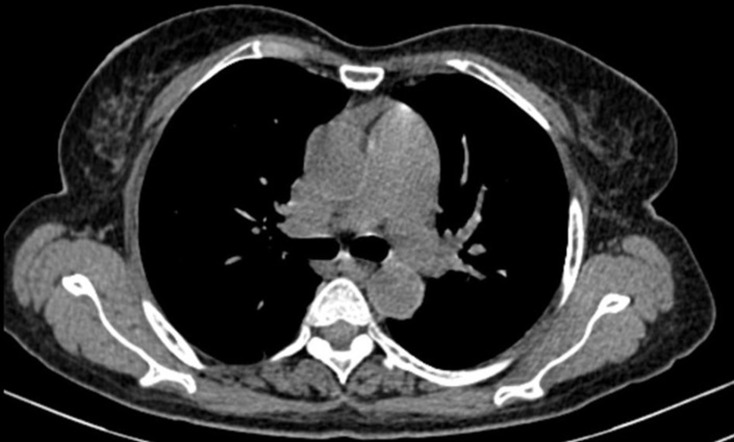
preoperative transverse section of chest computed tomography in lung showing no evidence of metastasis
